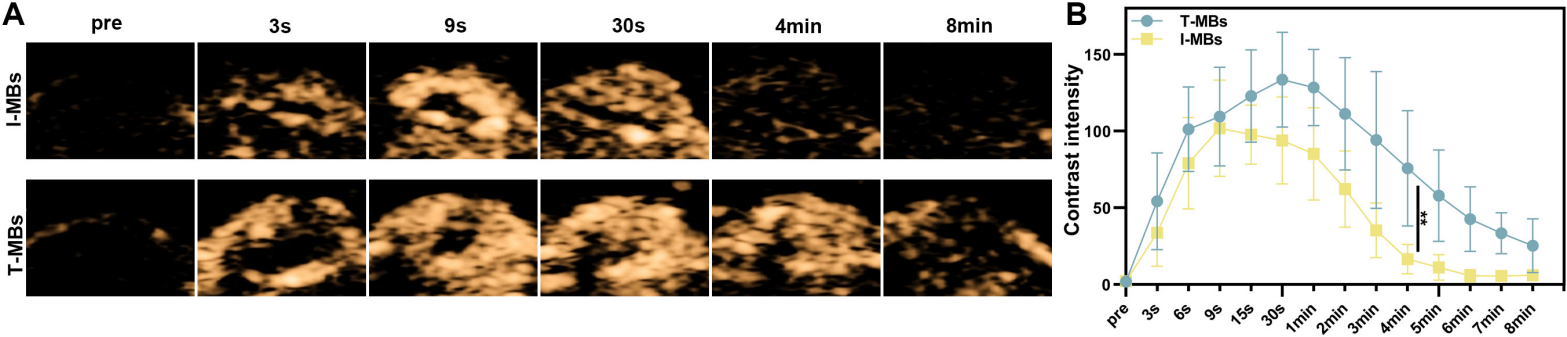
In vivo ultrasound molecular imaging of tumors. (A) Ultrasound molecular imaging of tumors with T‐MBs and I‐MBs in vivo. (B) Grayscale quantitative analysis of A, respectively (*n* = 5, *p* < 0.01).
